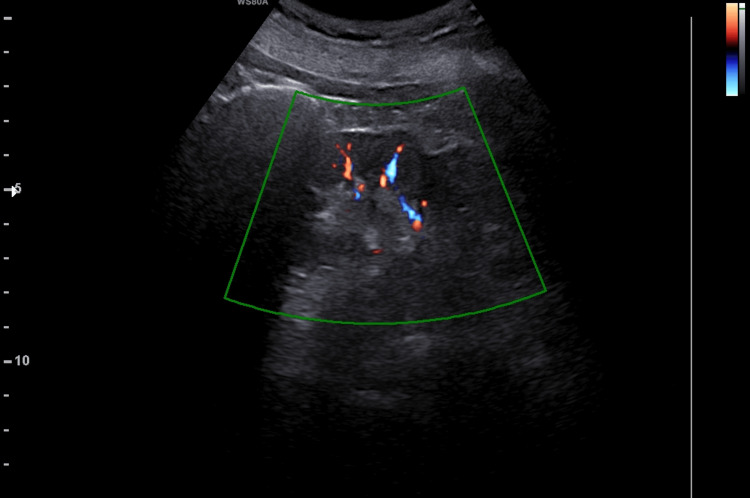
Postoperative ultrasound imaging of the left kidney (our case) The absence of the preoperative dilatation of the pelvicalyceal system and the presence of adequate perfusion of the renal parenchyma is evident.
